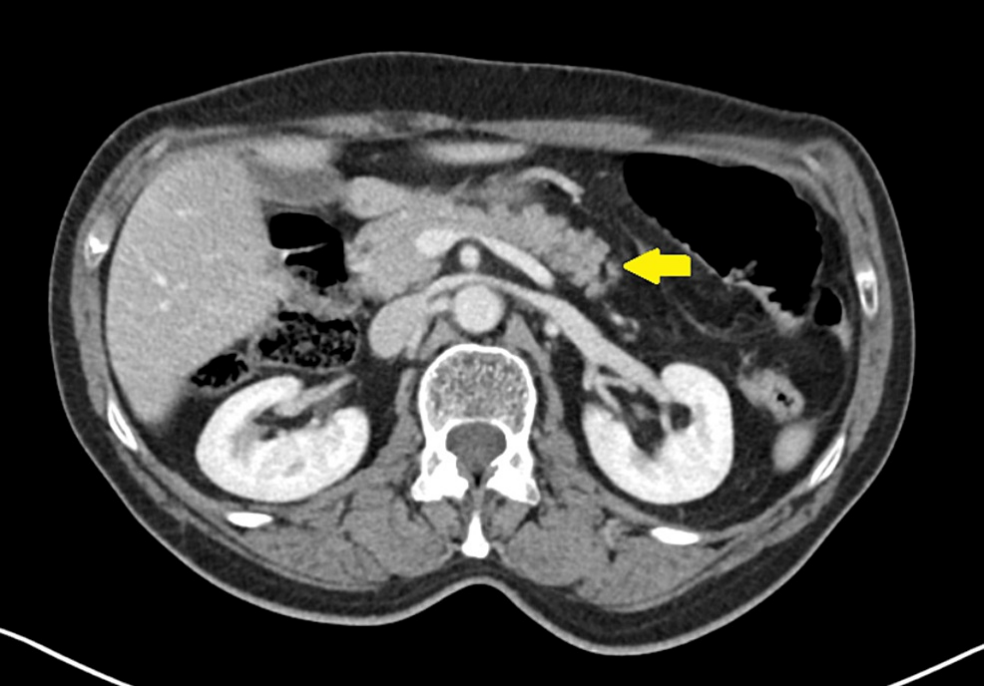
Abdominal computed tomography with contrast demonstrating pancreas without abnormalities (yellow arrow).
